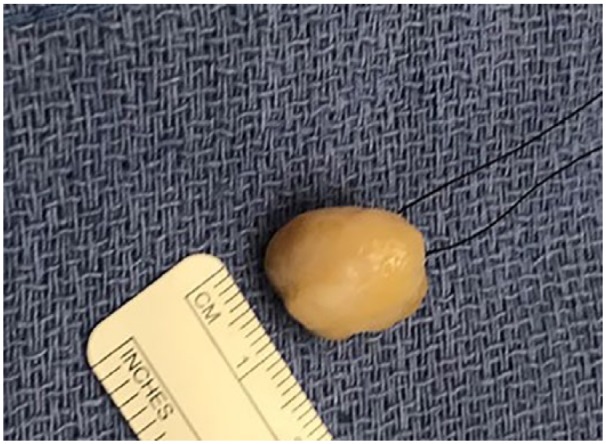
Schwannoma mass with size matching the measures in MRI.
